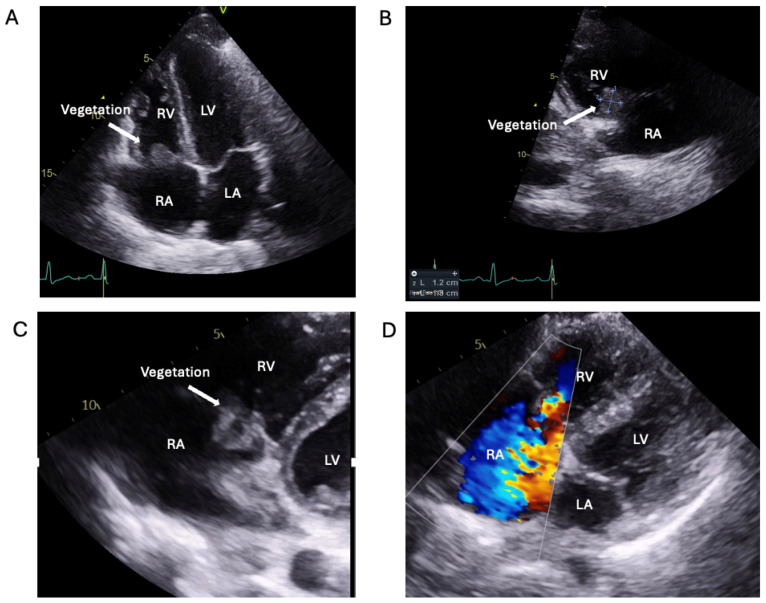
Transthoracic echocardiography of the right-sided IE of non-compliant high-risk young PWID with relapsing IE. (A): A4CV showing TV vegetation. (B): Modified parasternal long-axis view showing RV inflow and TV vegetation. (C): Modified parasternal long-axis view showing RV inflow and TV vegetation, after readmission post self-discharge. (D): Colour Doppler image of modified parasternal long-axis view showing RV inflow—Tricuspid Regurgitation (TR) after readmission. RV: right ventricle; LV: left ventricle; RA: right atrium; LA: left atrium.
